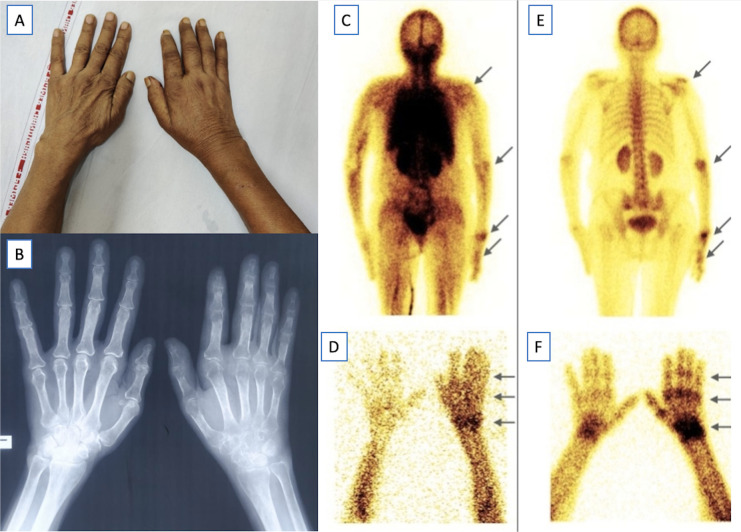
Diffuse swelling over right hand and forearm along with sparse hair (A), X-ray bilateral hands showing patchy osteopenia around right wrist and small joints of hand (B), ^99m^Tc-methylenediphosphonate (MDP) three phase bone scan: Blood pool phase images of whole body posterior view (C) and regional hand static dorsal view (D) showing relatively increased pooling of the tracer in the small joints of the right hand, right wrist, right elbow and right shoulder joint regions (arrows) compared to the left side; Delayed phase images of whole body posterior view (E) and regional hand static dorsal view (F) showing relatively increased uptake of the tracer (osteoblastic activity) in the periarticular regions of small joints of the right hand, right wrist, right elbow and right shoulder joint (arrows) compared to the left side. Image findings are suggestive of complex regional pain syndrome (CRPS) involving the right upper limb.
